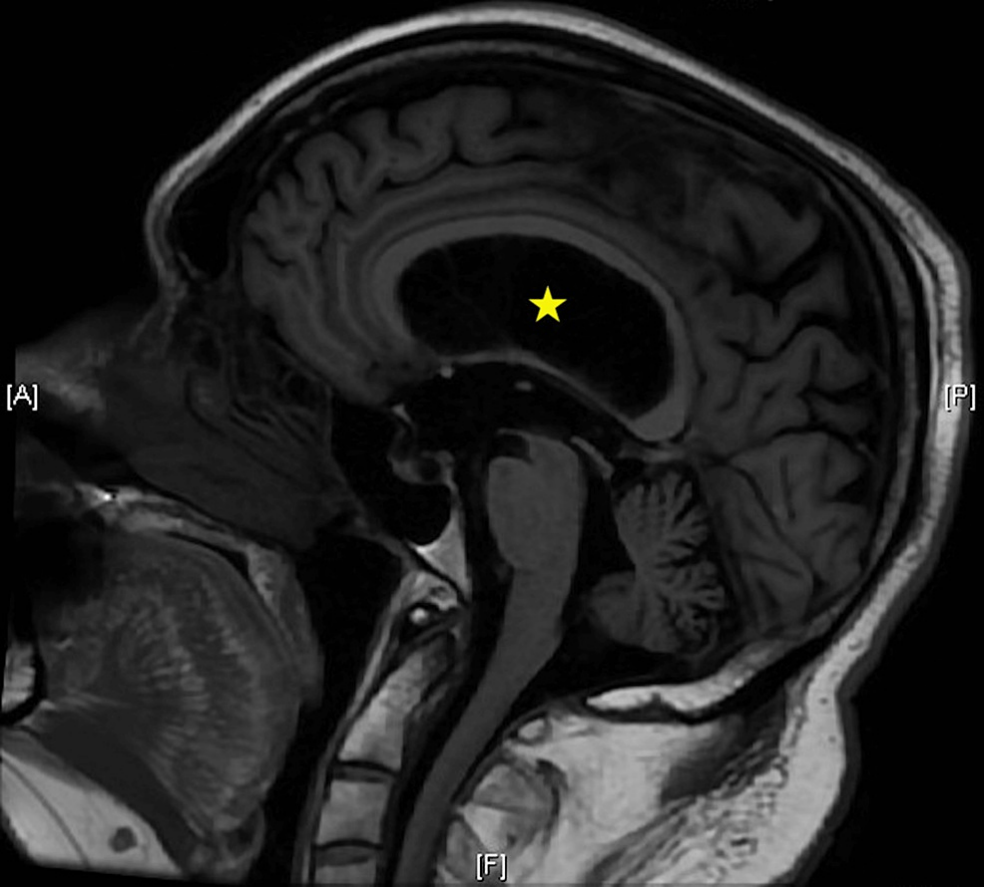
Magnetic Resonance image saggital view demonstrating interval improvement in hydrocephalus (star).
